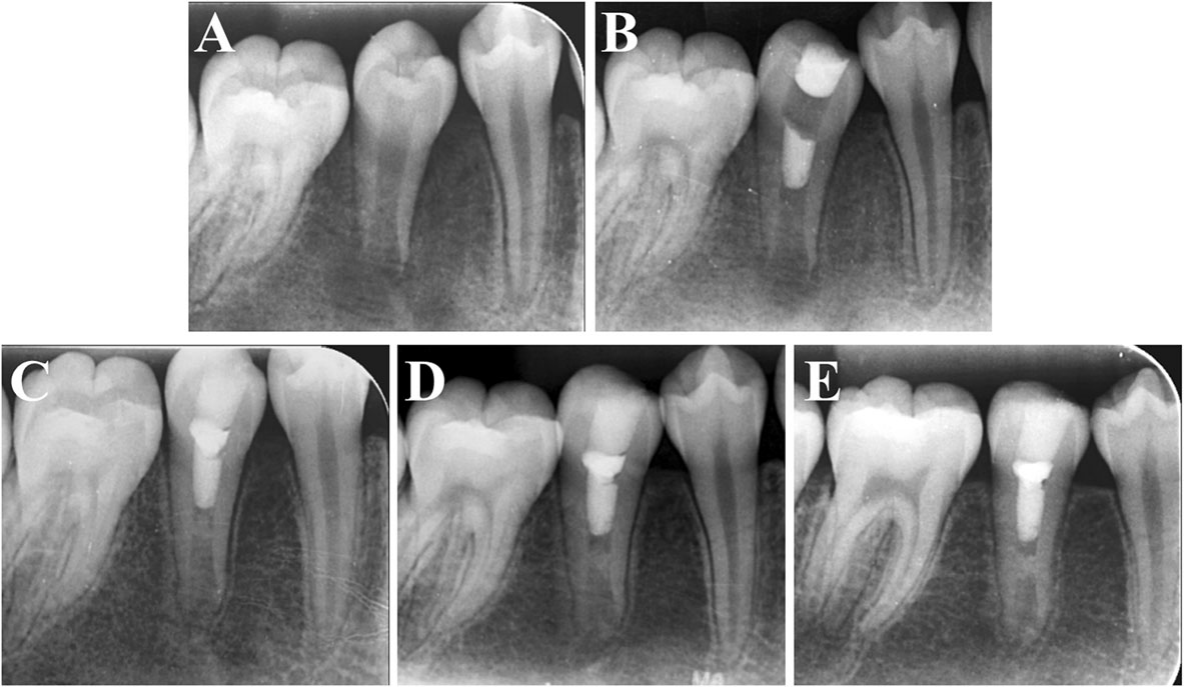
a An intraoral periapical radiograph of a 12-year-old girl showing a wide-open apex of #45 with thin lateral dentinal walls and apical radiolucency. b Three-month follow-up periapical radiograph of tooth #45 after undergoing conventional RET. c Six-month follow-up radiograph. d Nine-month follow-up radiograph. e Twelve-month follow-up radiograph showing apical radiolucency resolution, apex closure, root elongation and lateral dentinal wall thickening
